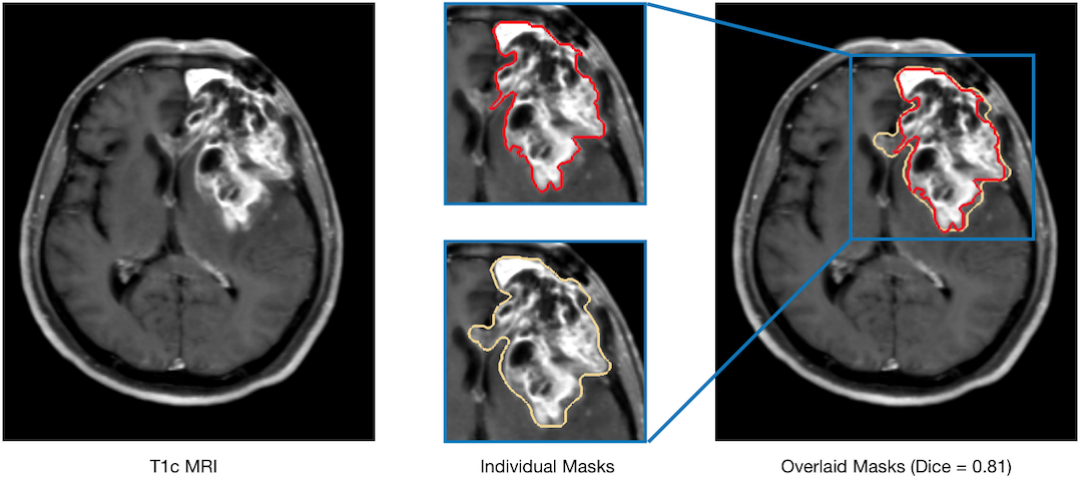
A T1c tumor image and its corresponding tumor masks created by two neuroradiologists independently, which shows the disagreement between annotators. MRI: magnetic resonance imaging; T1c: gadolinium-contrast-enhanced T1-weighted MRI.
